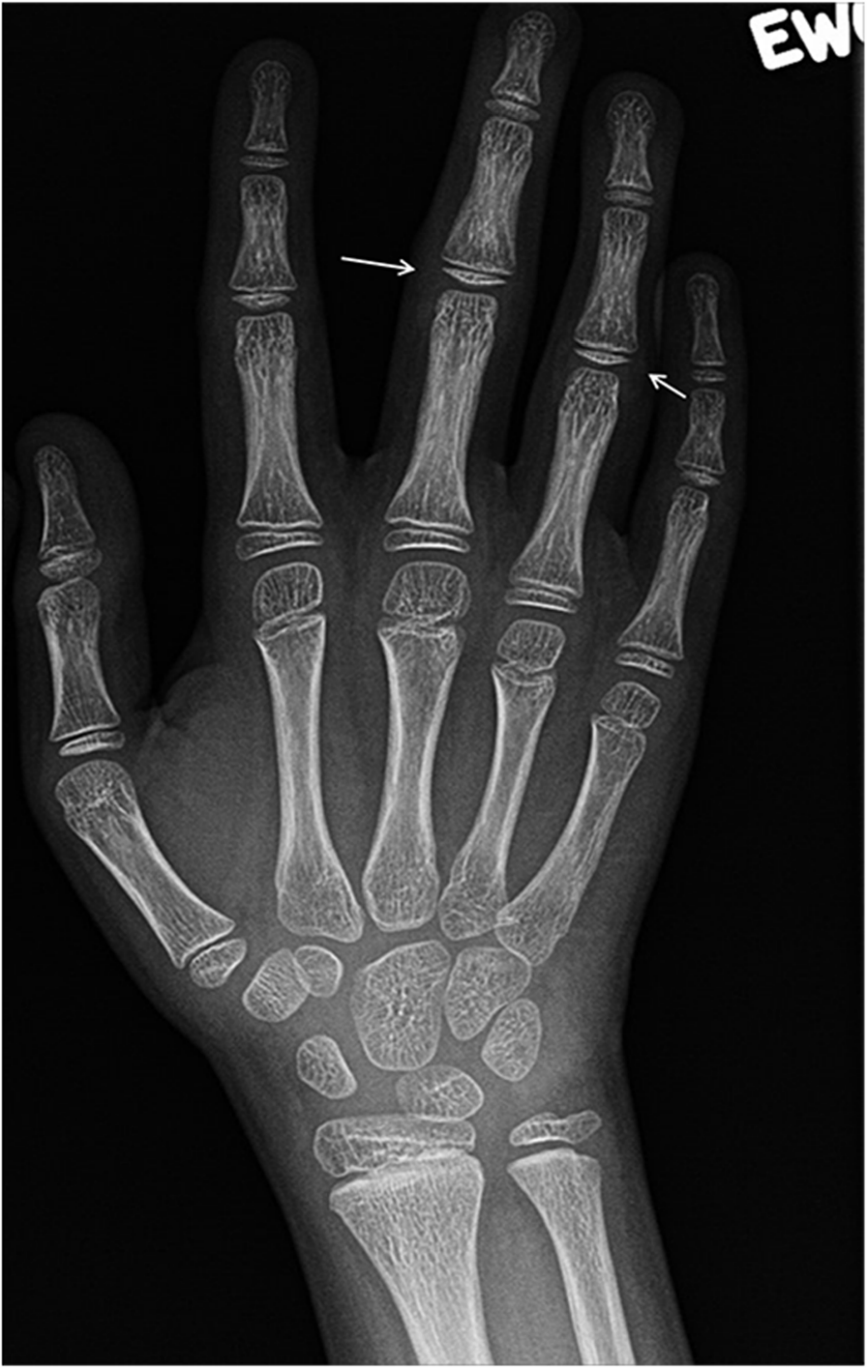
A 13-year-old girl with Aicardi-Goutieres syndrome. She has long history of arthritis, nodular rash, and chilblains started at the age of 2 years. Anteroposterior radiograph of the right hand demonstrates diffuse periarticular osteopenia, soft tissue swelling of the proximal interphalangeal joints (PIPs), mainly the third and fourth PIPs (arrows). There are no erosions, joint space narrowing or soft tissue calcifications. Similar changes are present in the left hand (not shown)
